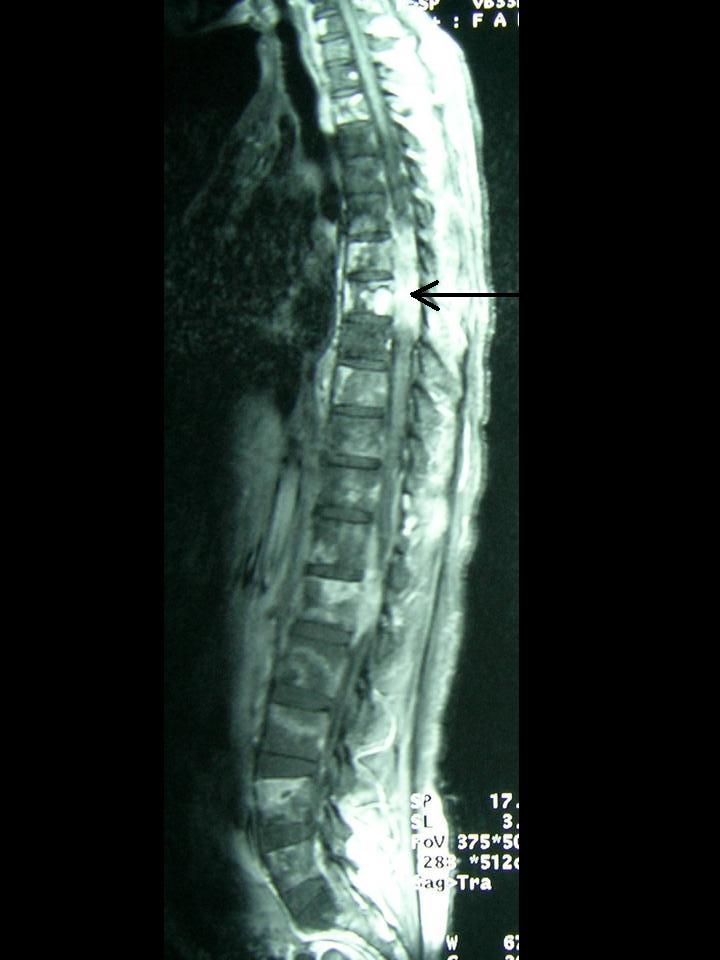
A contrast-enhanced T1 weighted sagittal MRI of the spine demonstrating multiple enhancing lesions in the vertebral bodies in keeping with bony metastases and cord compression at T7 level (arrow).
